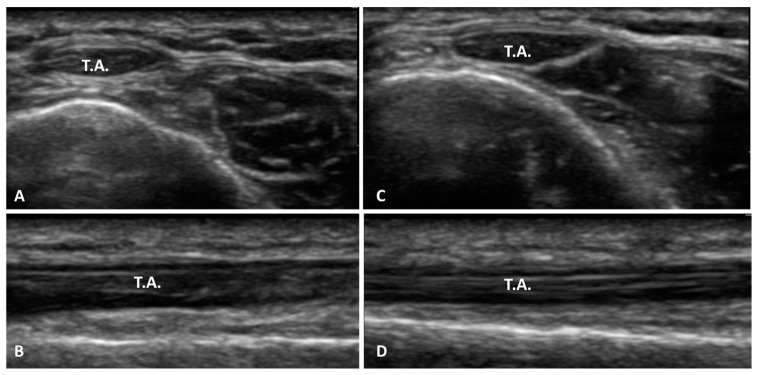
Ultrasound images of SEAR thickness: (A) healthy side group 1: transversal axis; (B) healthy side group 1: longitudinal axis; (C) sprain side group 1: transversal axis; (D) sprain side group 1: longitudinal axis. T.A., tibialis anterior tendon.
